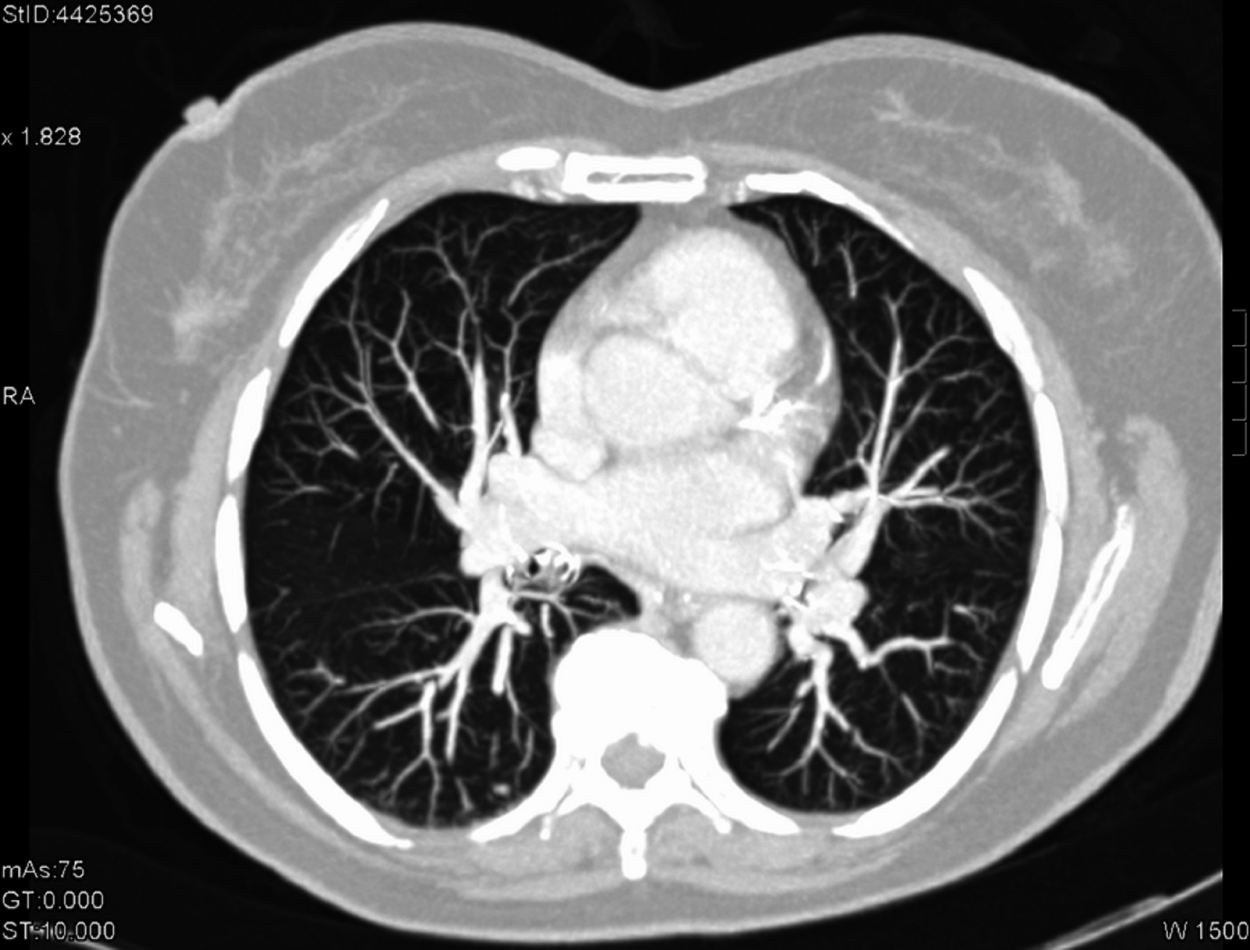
Patient 3: Computed tomography (CT) imaging reveals resolution of significant lung nodules and stability over eight years post initial treatment
